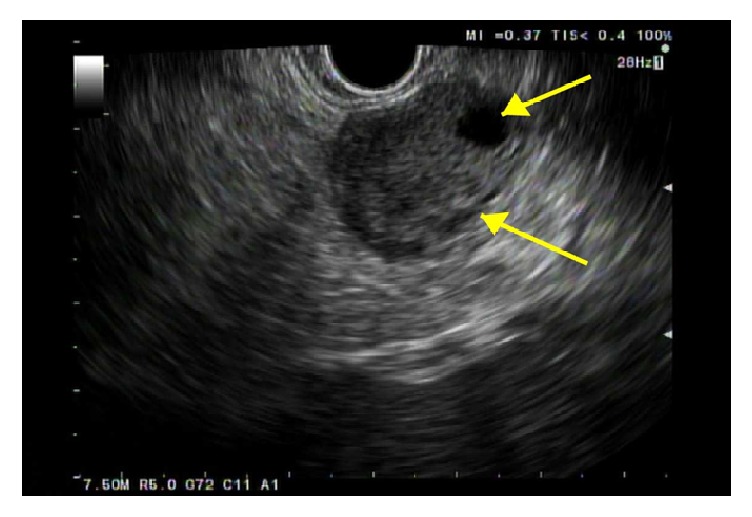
Solid mass with cystic component during an endoscopic ultrasound.
